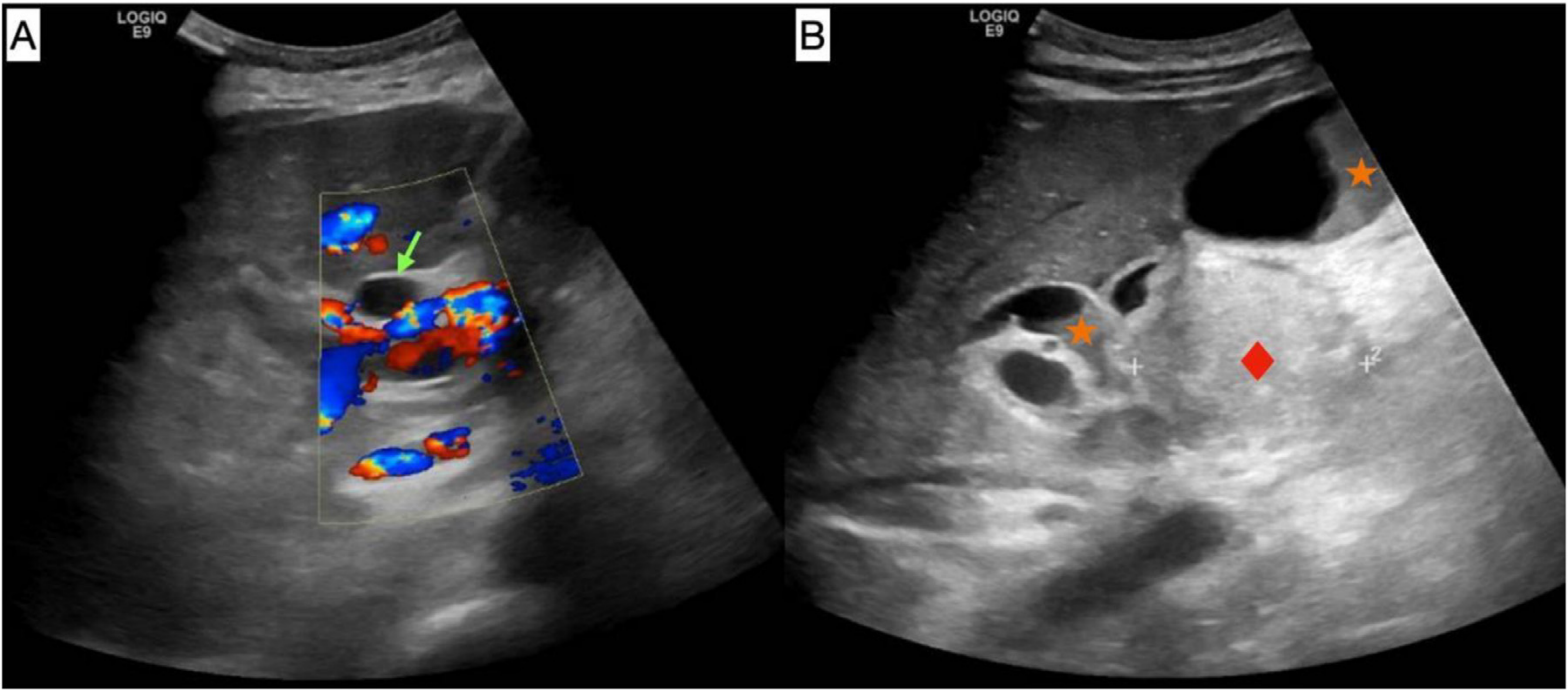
Selected transabdominal ultrasound images at the level of (A) Porta hepatis; (B) Pancreatic head. The common bile duct (CBD) (light green arrow) appears dilated, measuring approximately 0.9 cm, likely due to extrinsic compression by the inflamed and edematous pancreatic head (red diamond). This results in minimal intrahepatic biliary dilatation. Thick echogenic sludge is visualized within the gallbladder and distal CBD (orange stars).
